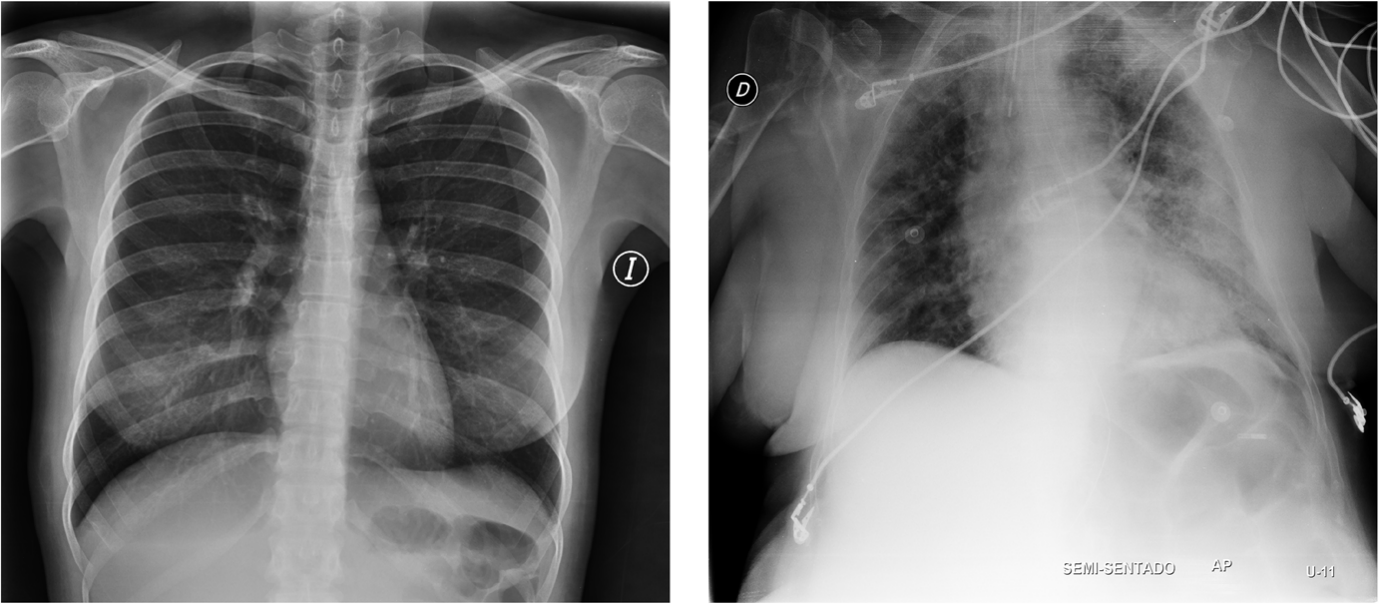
Resized chest radiographs of two patients from COVIDx8 [37]. Left side shows normal finding (COVID-19 negative) and right side shows severe pneumonia (COVID-19 positive)
